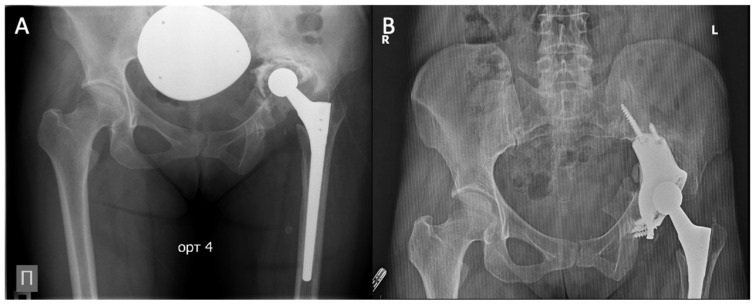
Radiographs of a 52-year-old female patient with a Paprosky type IIIB defect: (A) Preoperative; (B) 12 months after reconstruction with a custom-designed additive-manufactured acetabular component.
